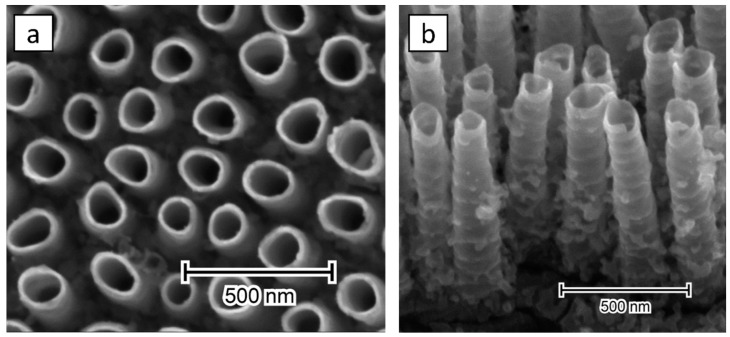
(a) Top and (b) side SEM image of the TiO_2_ nanotubes after ultrasonic-treatment in a nickel bath.
